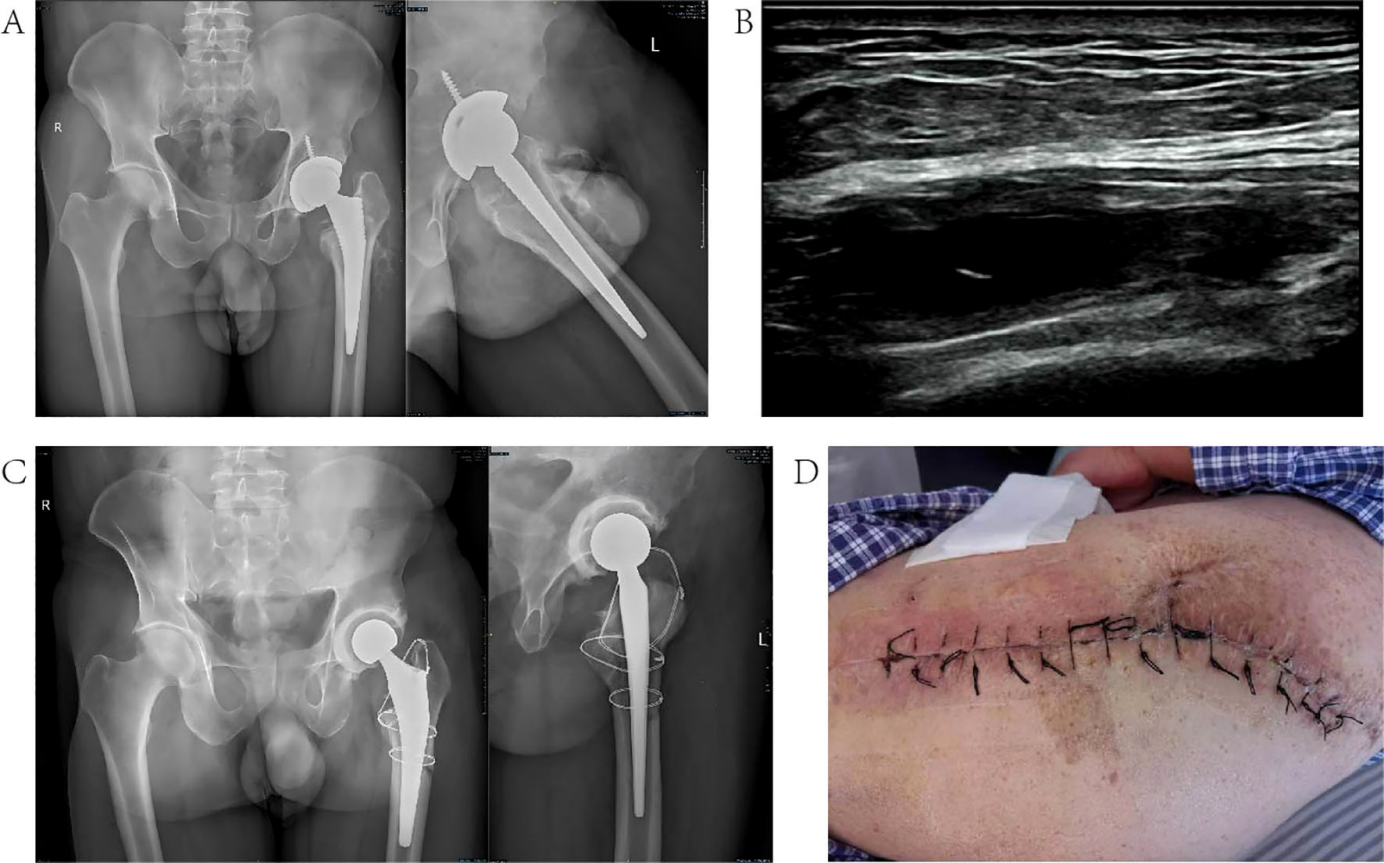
Typical case 1. (A) Frontal and lateral radiographs of the hip joint before the one-stage revision surgery; (B) localized ultrasound of the hip joint; (C) frontal and lateral radiographs of the hip joint after the revision surgery; (D) good healing of the incision without signs of infection was seen at the time of discharge from the hospital.
